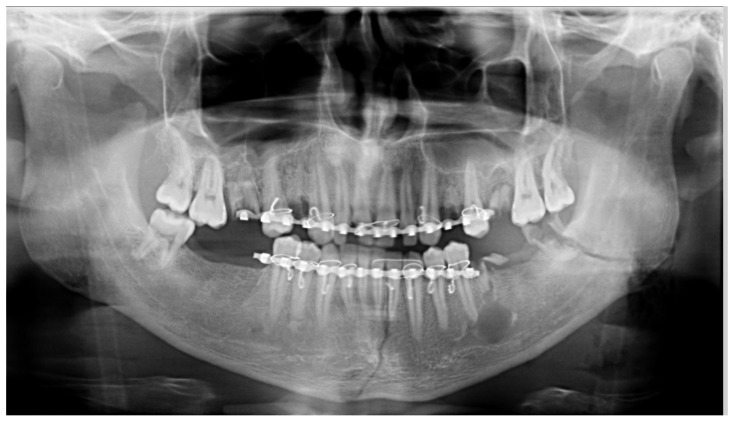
Orthopantomography in a patient with a double mandible fracture presents a positioning error, with lateral rotation of the head towards the left side and, consequently, an obvious asymmetry in the image. The errors are caused by the impossibility of using the bite-block when applying bimaxillary immobilization.
